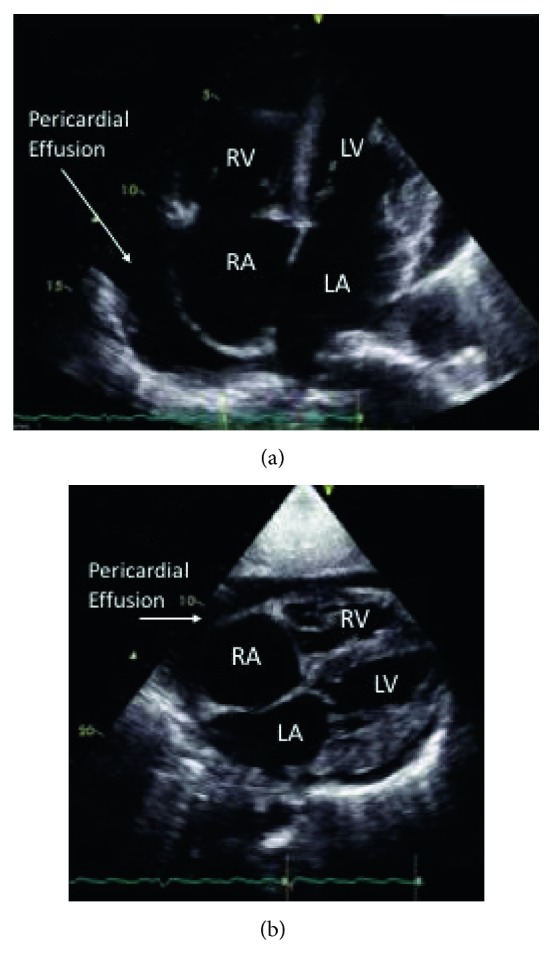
Transthoracic ultrasound on admission: (a) apical four-chamber view and (b) subcostal four-chamber view showing a large pericardial effusion and dilated cavities. On Doppler and dynamic views, global hypokinesis and severe mitral regurgitation were appreciated (RA = right atrium; RV = right ventricle; LA = left atrium; LV = left ventricle).
